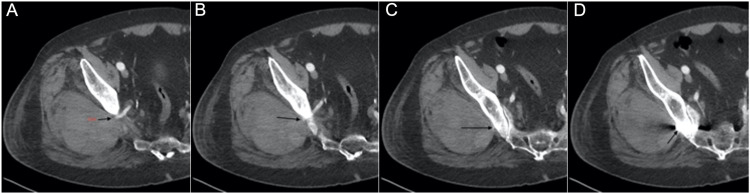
Computed tomography angiography of right lower extremity. A series of axial cuts from caudal to cranial, A to D, showing the superior gluteal artery (black arrow) exiting the greater sciatic foramen and traveling superiorly to end at the side of the implants.
